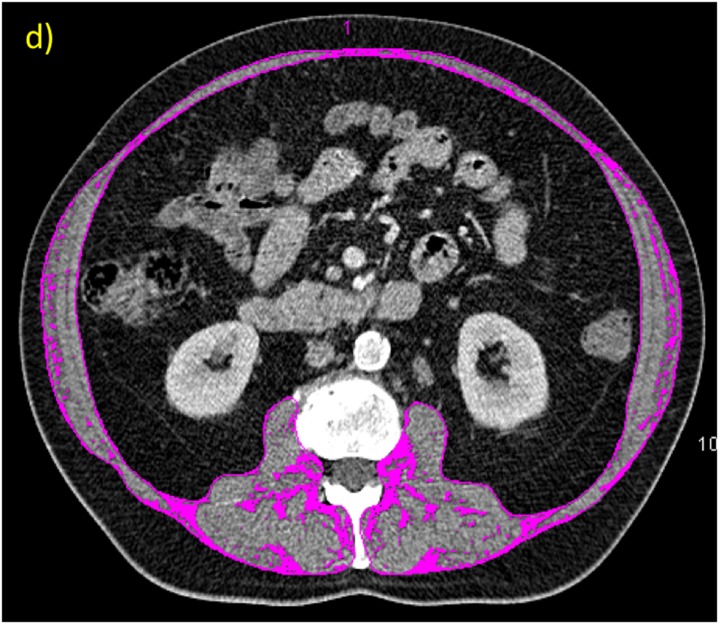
Example of densitometric quantification of adipose tissue area (threshold: -190 to -30 HU) in a CT scan at level L3/4: ROI determining TAT (a) and VAT (b). Example of densitometric quantification of muscle area in a CT-scan at level L 3/4 with ROI determining muscle compartments with threshold: -29 to +150 HU (c) and IMFA with threshold: -190 to-30HU (d).
